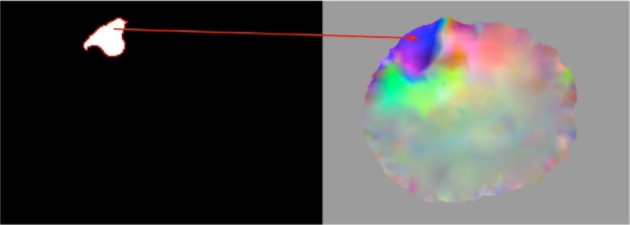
Representation of the map between the corrected region of the warped pre-op segmented MRI (left) and the image deformation field DF_addi_ (right).
